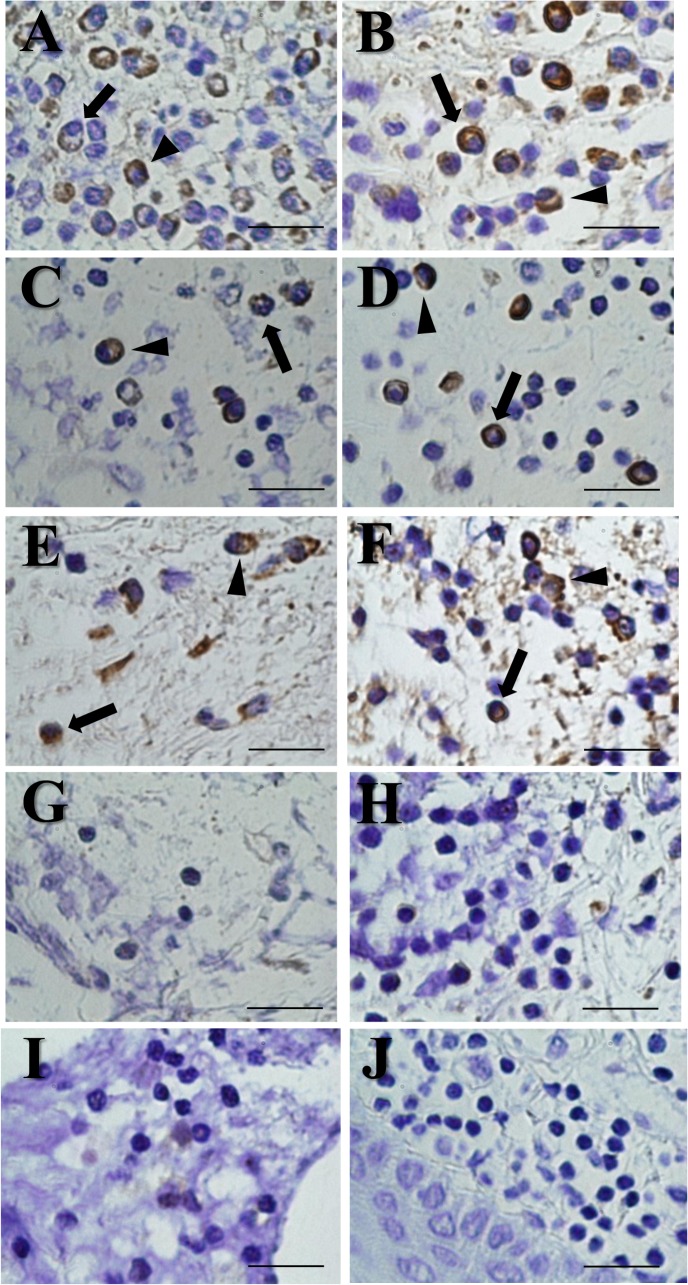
Detection of EBER using *in situ* hybridization. (A-F) *In situ* detection of EBER-expressing cells in periapical granulomas (6 out of 9) was shown in the cytoplasm and nuclei of B cells (arrows) and plasma cells (arrow heads). (G-I) Three periapical granulomas showed EBER-negative expression. (J) Healthy gingival tissues never showed positive expression. Scale bar = 50μm.
